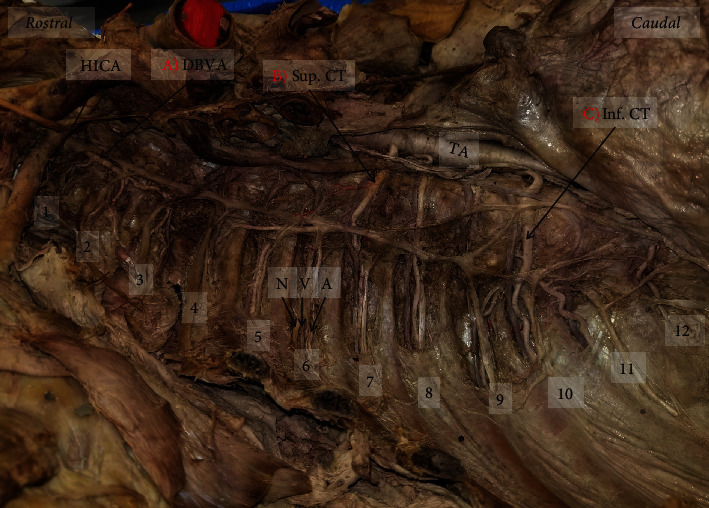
Right posterior thoracic wall with exposed intercostal spaces: (a) the descending branch of the vertebral artery supplying the 1st-3rd ICS; (b) superior common trunk arising from TA between7th and 8th thoracic vertebrae supplying 4th-7th ICS; (c) inferior common trunk arising from TA between 10th and 11th thoracic vertebrae supplying 9th-11th ICS. Numbers 1-11: ICS number; 12: subcostal space; HICA: highest intercostal artery; DBVA: descending branch of the vertebral artery; Sup. CT: superior common trunk; TA: thoracic aorta; Inf. CT: inferior common trunk; N: nerve; V: vein; A: artery.
